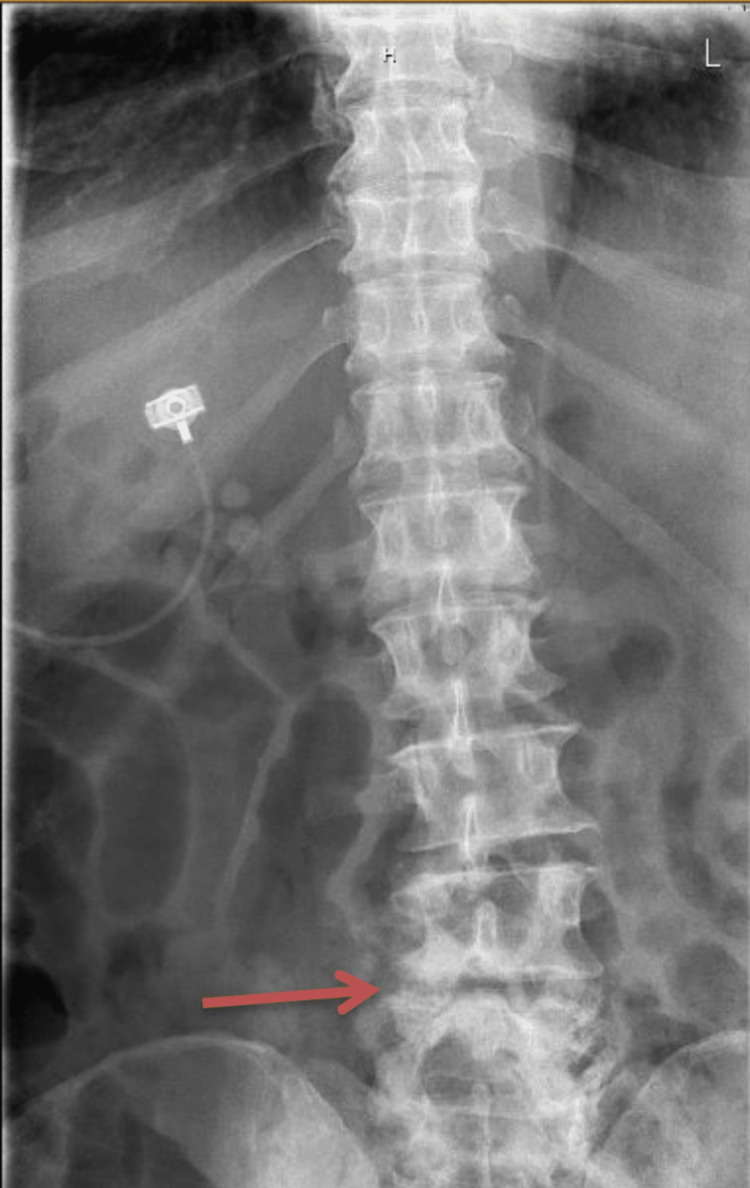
X-ray of the lumbar spine (anteroposterior view): posterolisthesis of L4-L5 (red arrow).
